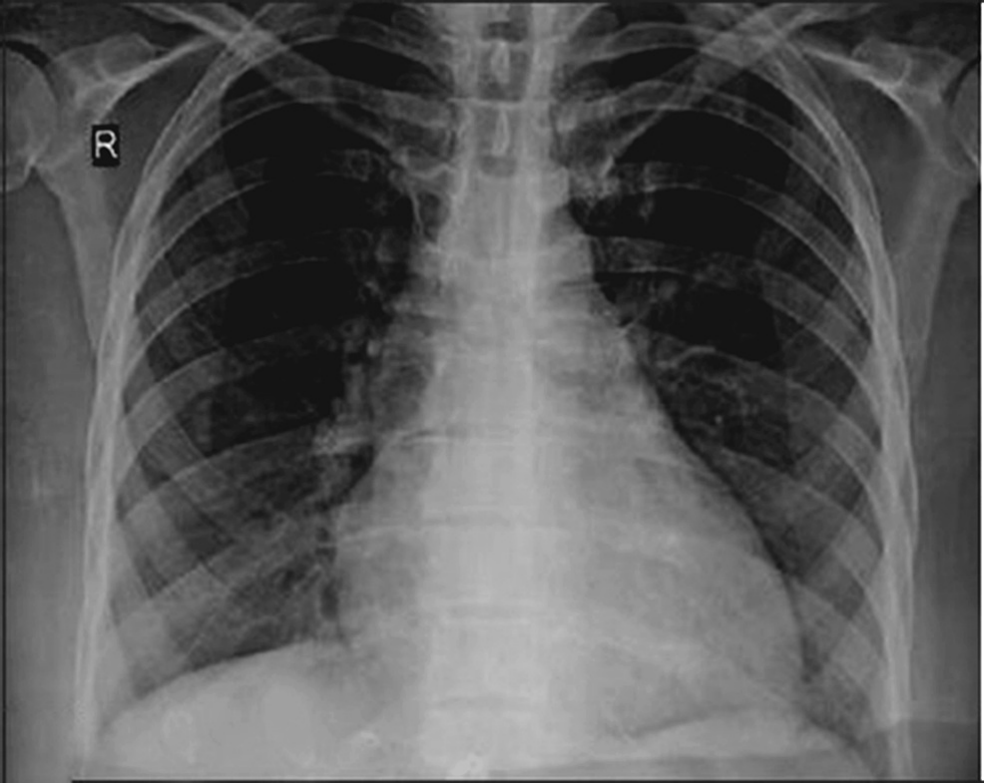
X-ray of the chest showing severe cardiomegaly.
